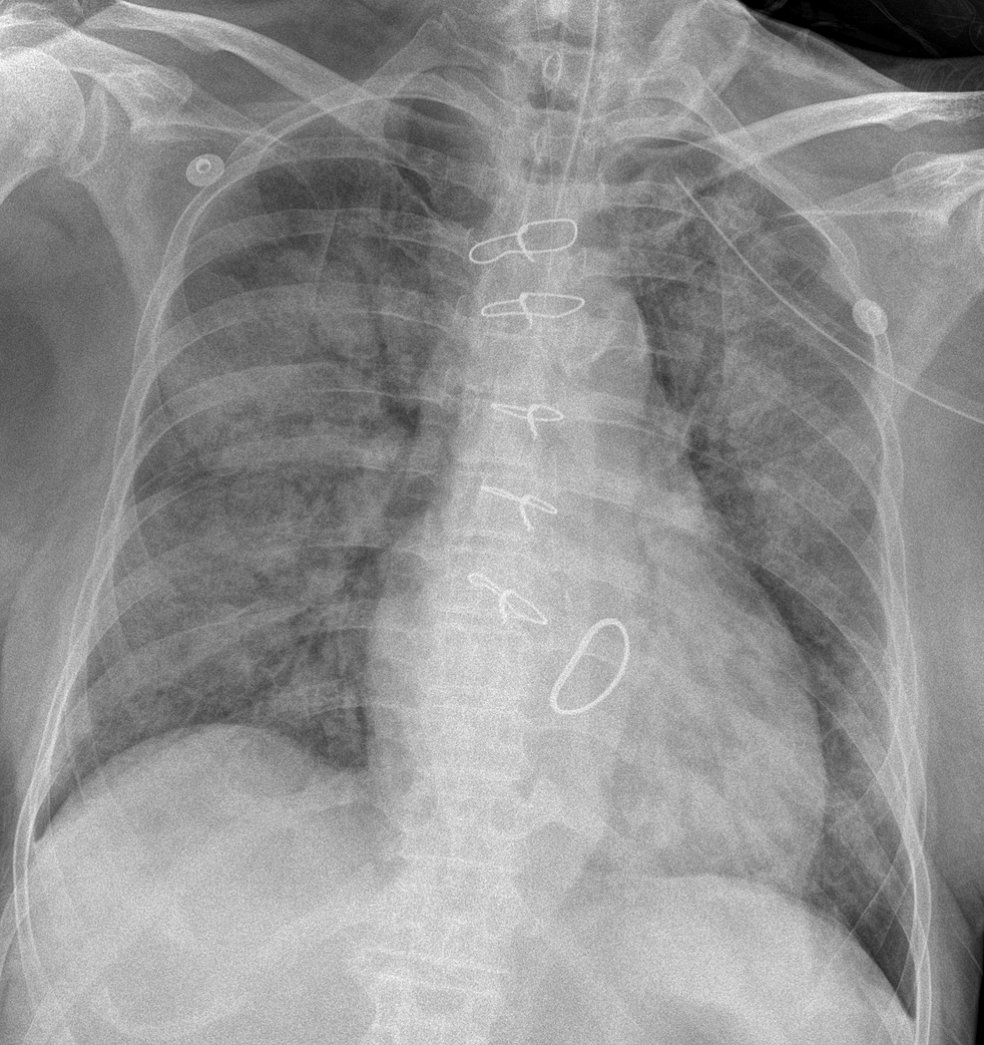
Atypical radiographic pattern in a critical SARS-CoV-2 pneumonia case The CXR showed diffuse consolidations in bilateral lung fields with the air bronchogram sign. The Brixia score was 18, the RALE score was 8, and the mCXR score was 12. This case was classified as a critical situation based on clinical severity. The qCSI was 10.
